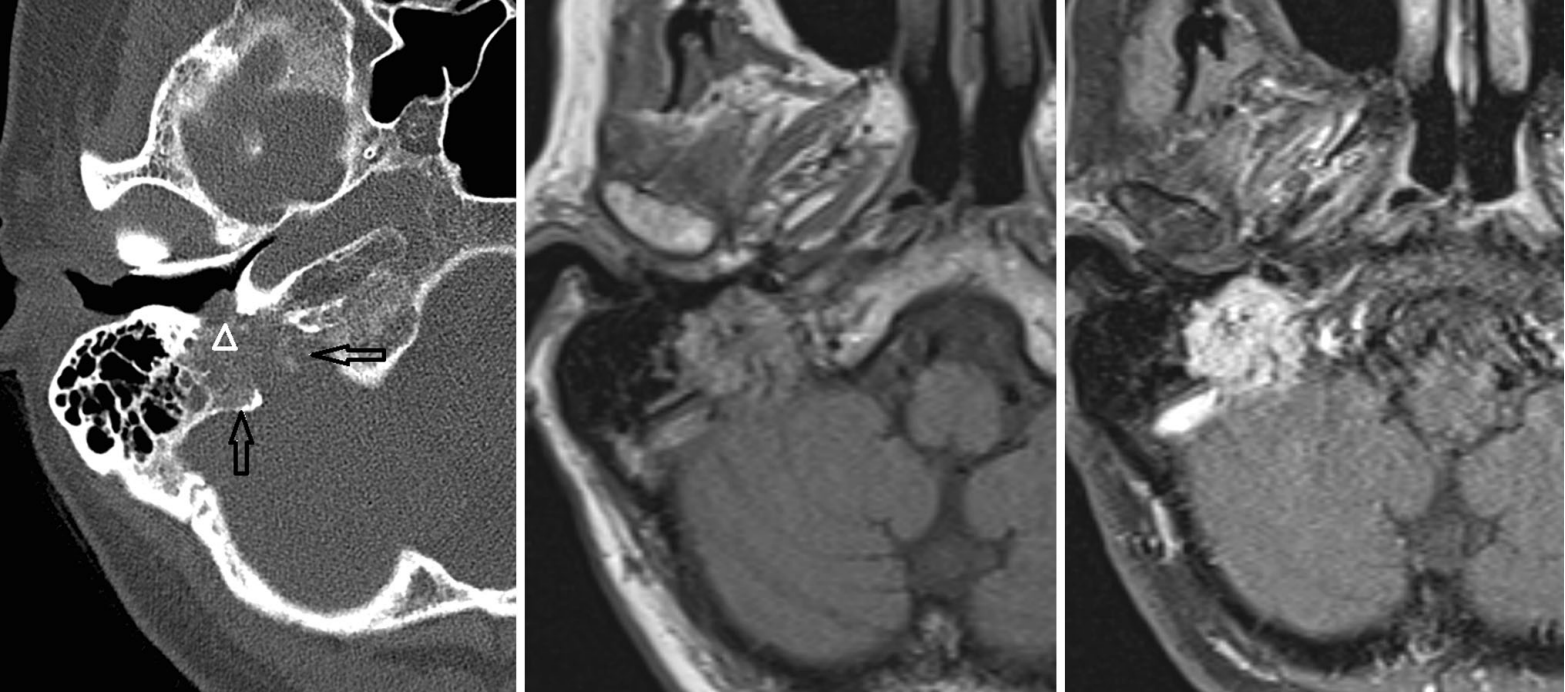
Glomus jugulotympanicum on CT and MRI. *Left* CT demonstrating erosive changes at the jugular bulb (*arrows*). Notice extension of the soft tissue into the middle ear (*arrowhead*). *Middle* Axial T1-W image shows a mixture of signal intensities due to vascular flow voids, which makes up the ‘salt and pepper’ appearance. *Right* Axial contrast-enhanced T1-W with fat suppression demonstrates avid contrast enhancement of the tumor
